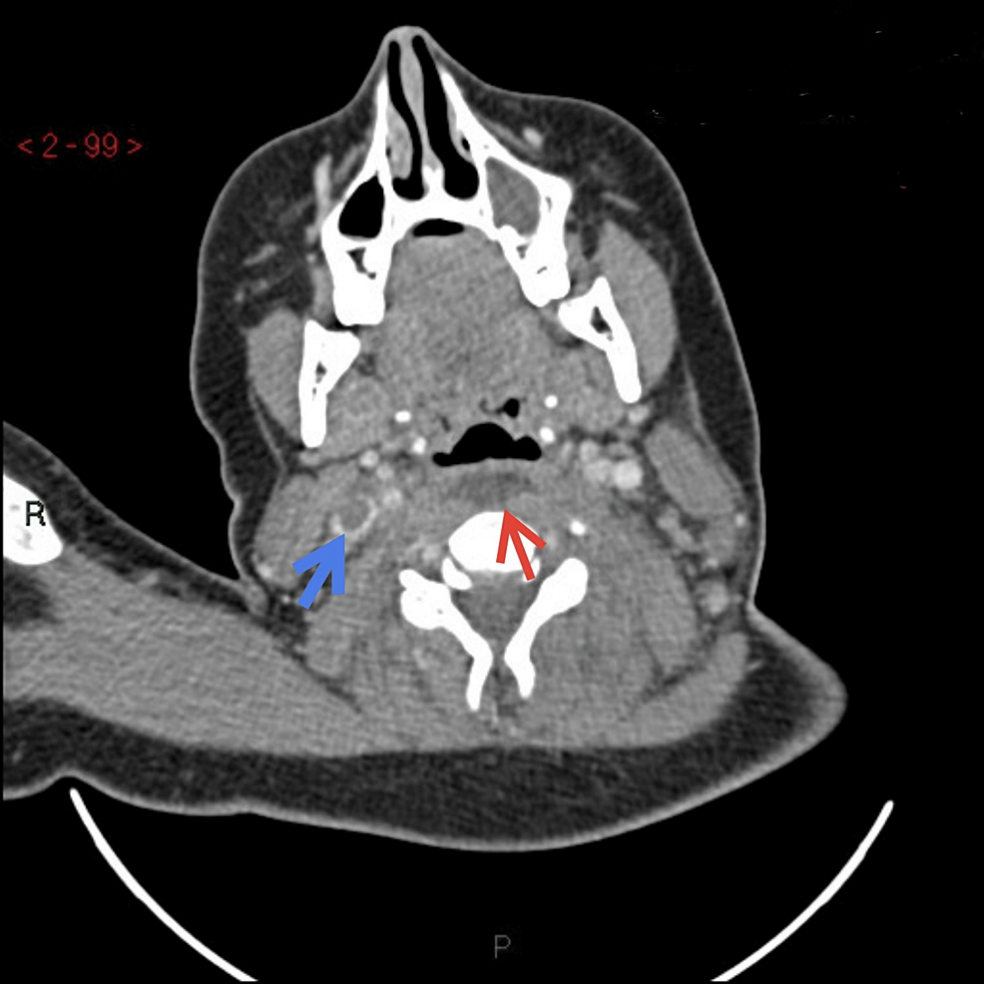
CT contrast study of neck transverse view. The blue arrow indicates the right internal jugular vein thrombus. The red arrow indicates retropharyngeal fluid suspicious of abscess formation.
